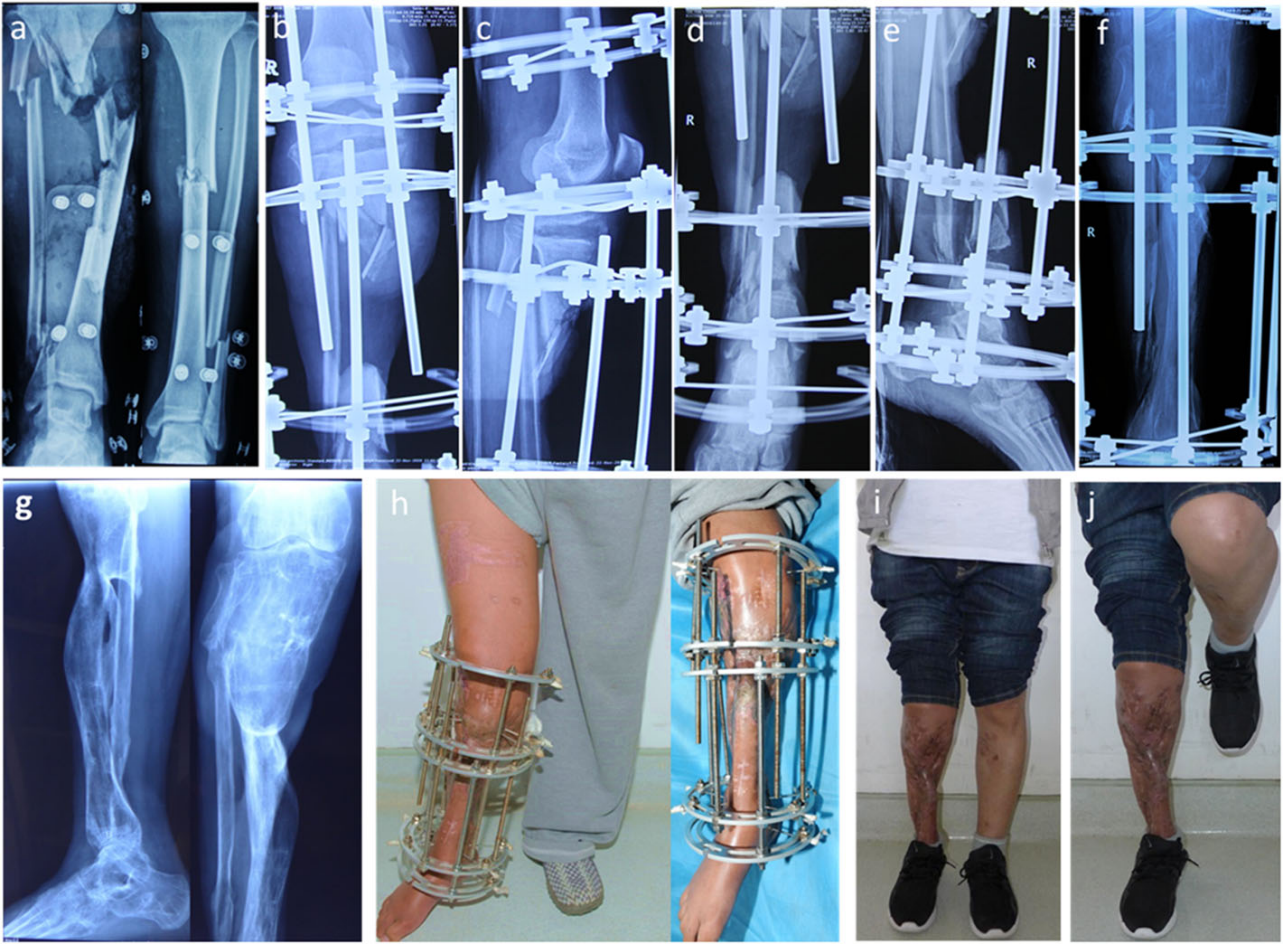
a Radiograph of case no. 31 who was involved in a traffic accident resulting in right tibia open fracture and Gustilo IIIB. b-e After three previous debridement operations, there was a defect of 18.2 cm on the tibia, and the proximal and distal osteotomy was performed. The osteotomy sites were obviously shown on the X-rays. f Radiograph of transport bone segment reaching docking site 30 months after osteotomy. g Union was achieved both in docking site and elongation area 35 months post-operatively. h Soft tissue defect was closed during the process of bone transport 5.8 months after osteotomy. i-j Complete bone and soft tissue union was achieved and frame was removed 35 months after osteotomy
